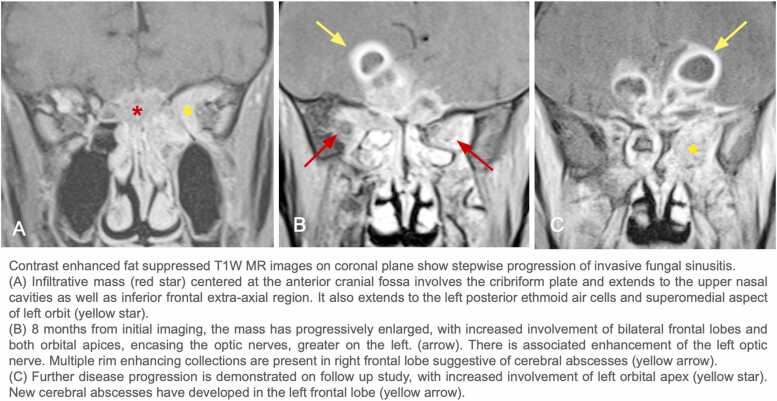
Contrast enhanced fat suppressed T1W MR images on coronal plane show stepwise progression of invasive fungal sinusitis.
